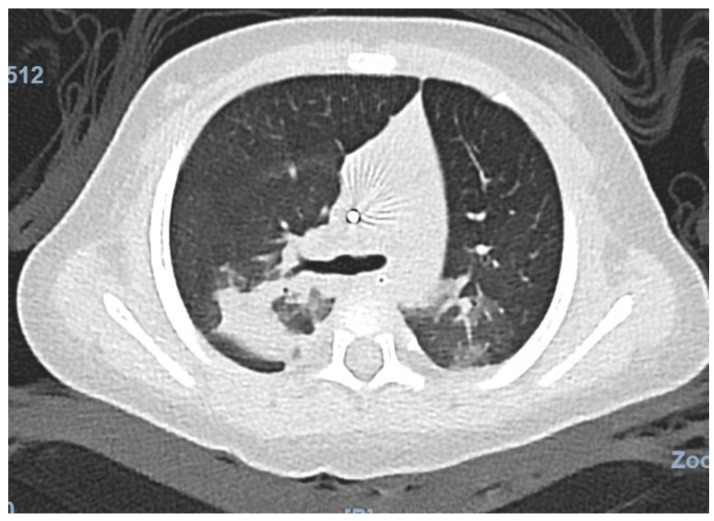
Chest computed tomography revealing bilateral pulmonary infiltrates.
